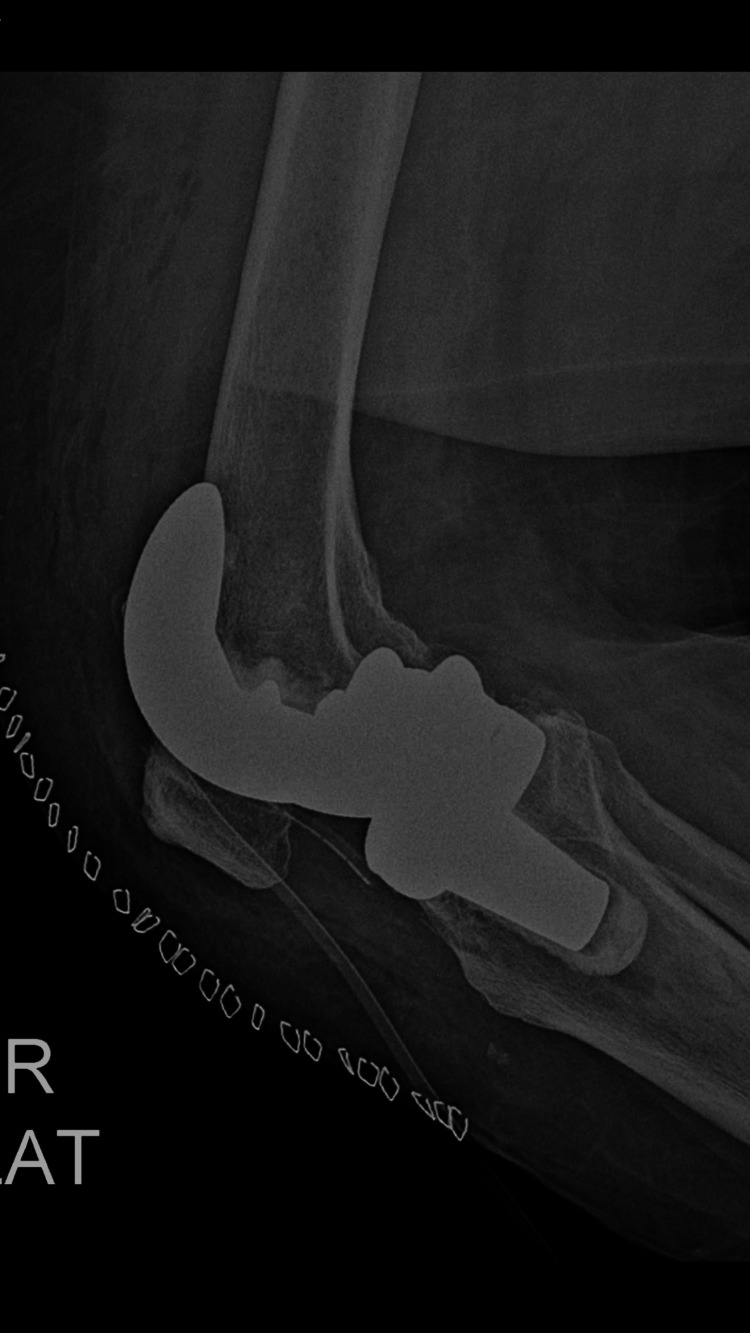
Immediate postoperative lateral radiograph of the right knee Immediate postoperative lateral radiograph of the right knee confirming appropriate placement and alignment of the femoral component following total knee arthroplasty.
